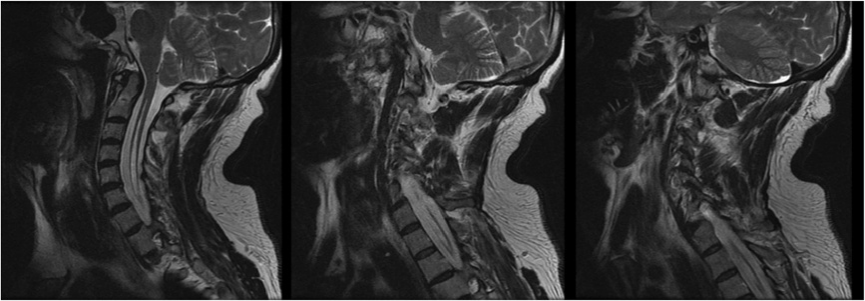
Magnetic resonance imaging of the cervical spine. Sequential T2 fast recovery fast spin echo sequence sagittal cuts demonstrating the syrinx from the level of C1 extending inferiorly into the thoracic spinal cord.
